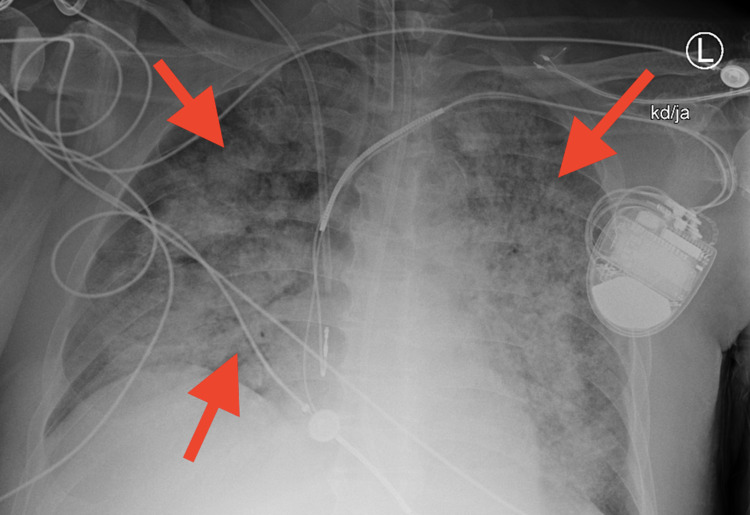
Chest X-ray showing near total atelectasis of the left lung, with interval increased bilateral opacities consistent with pulmonary hemorrhage (arrows)
